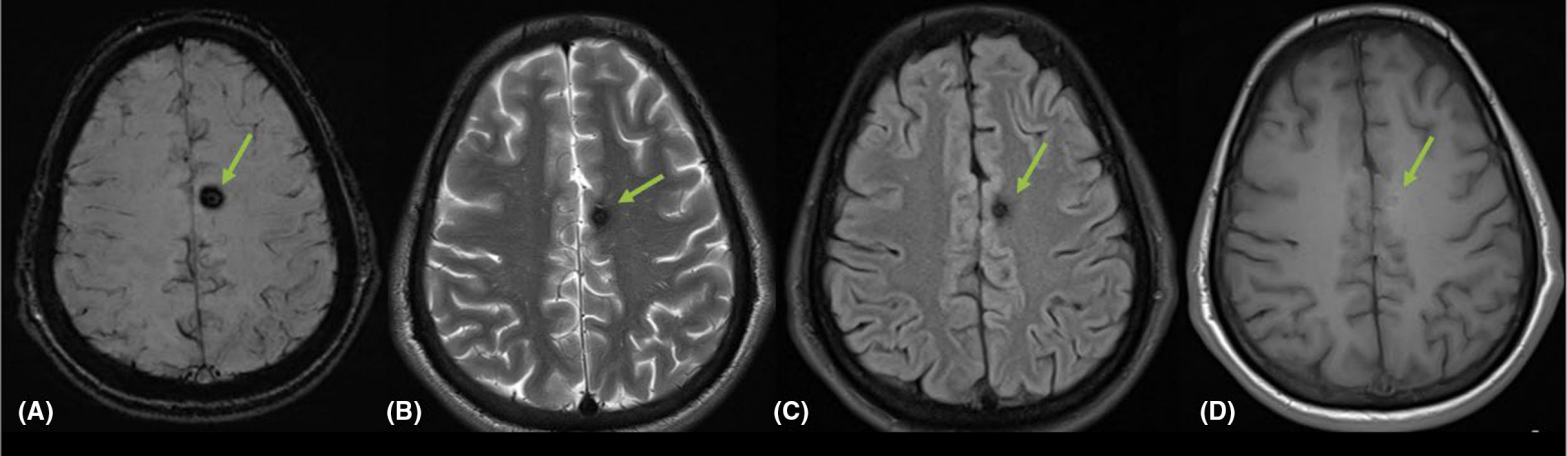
MRI head selected axial cuts: (A) SWI, (B) T2, (C) FLAIR and (D) T1, showed a focal area of significant SWI blooming measuring 5.5 mm in the left frontal parasagittal area (green arrows) that showed significant low T2, FLAIR, T1 signal intensity; features suggestive of cavernoma.
